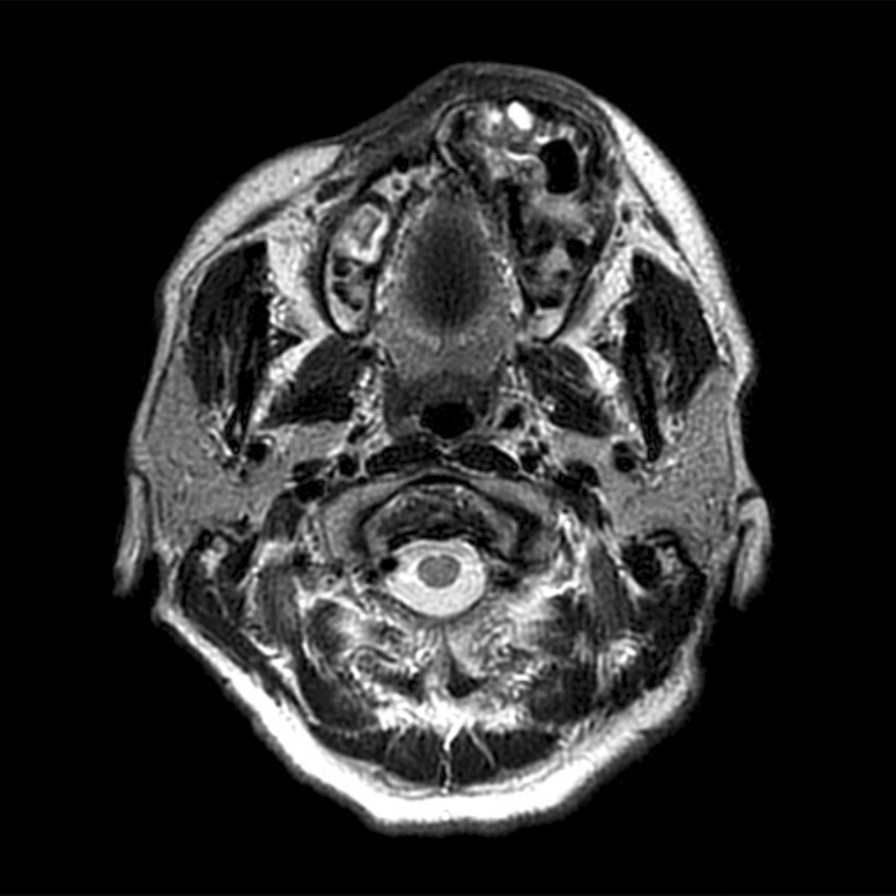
Magnetic resonance imaging findings immediately before surgery. Lesion with mixed low- and high-signals observed from the left alveolar ridge to left maxillary sinus on T2-weighted imaging was observed, without clear metastases to the surrounding tissues
